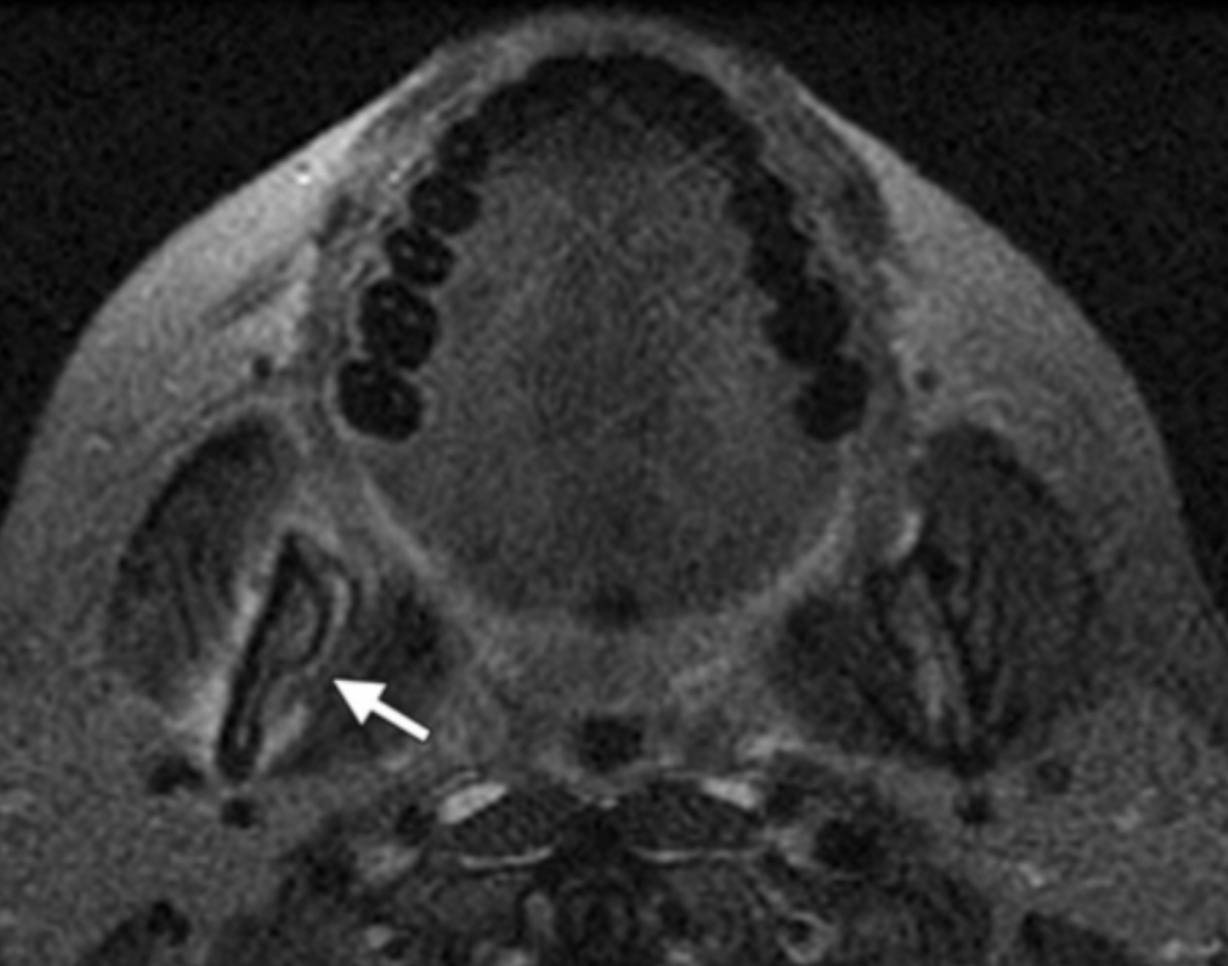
The appearance of ONJ on MRI modality: T2-weighted image Tissue window with increased signal intensity in the adjacent soft tissues of the right mandible (white arrow) ONJ: osteonecrosis of the jaw; MRI: magnetic resonance imaging
